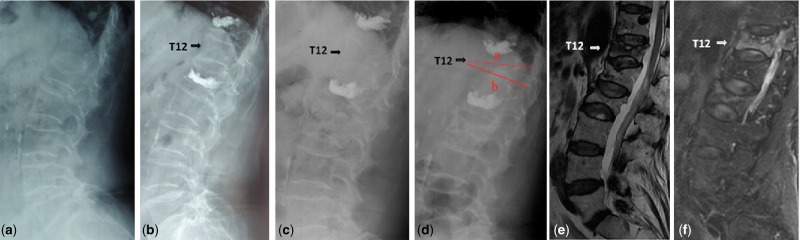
Images obtained in a 74-year-old woman with OVCFs. (a) The lumbar lateral radiograph before procedure. (b) T12 is a sandwich vertebral body located between L1 and T11 preciously treated with vertebral cement augmentation. (c) Follow-up after 12 months showed no loss of T12 height. (d) Patients felt back pain after 27 months after procedure. Lateral radiograph showed obvious compression within T12. (e) T2-weighted image indicated high signal intensity within T12. (f) Image showed high signal intensity within T12, indicating fresh vertebral fractures
